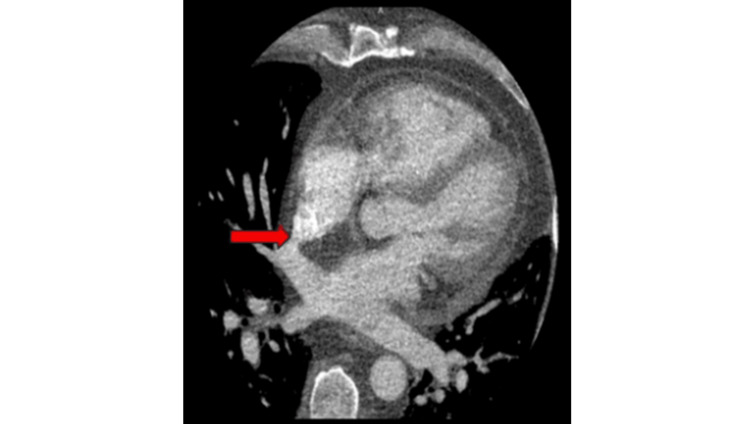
Cardiac computed tomography angiography Cardiac computed tomography demonstrated a partial anomalous pulmonary venous connection to the right atrium.
